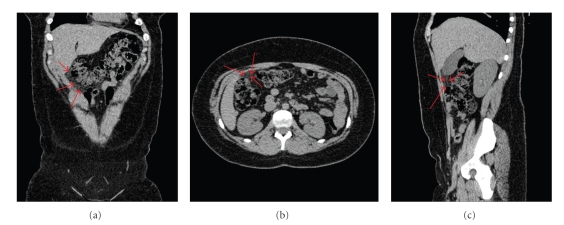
Unenhanced CT images (a) coronal, (b) axial, (c) sagittal show a focal area of hyperattenuating omental fat stranding (arrows).
